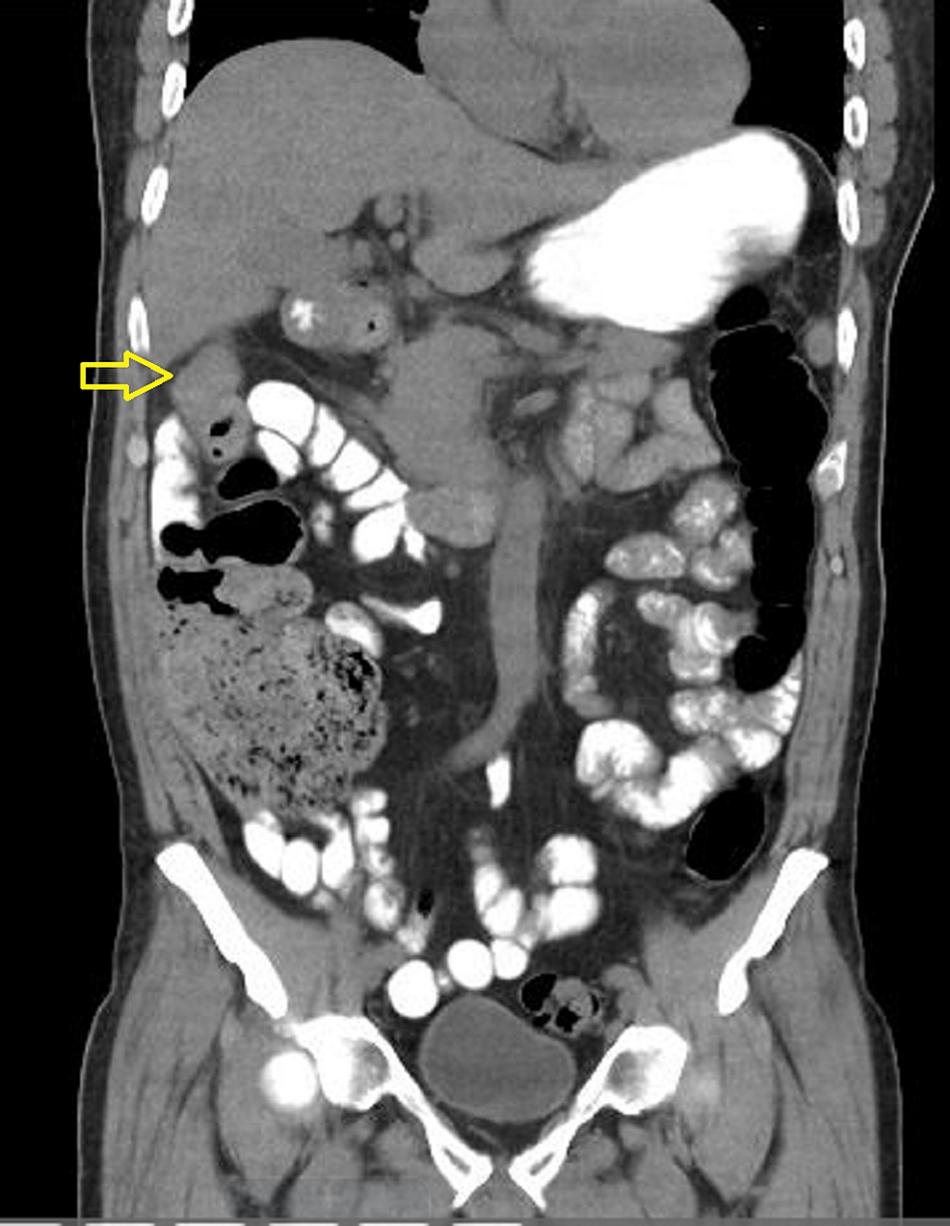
Coronal section of abdominal CT scan showing thickened hepatic flexure of the colon (marked with arrow)
